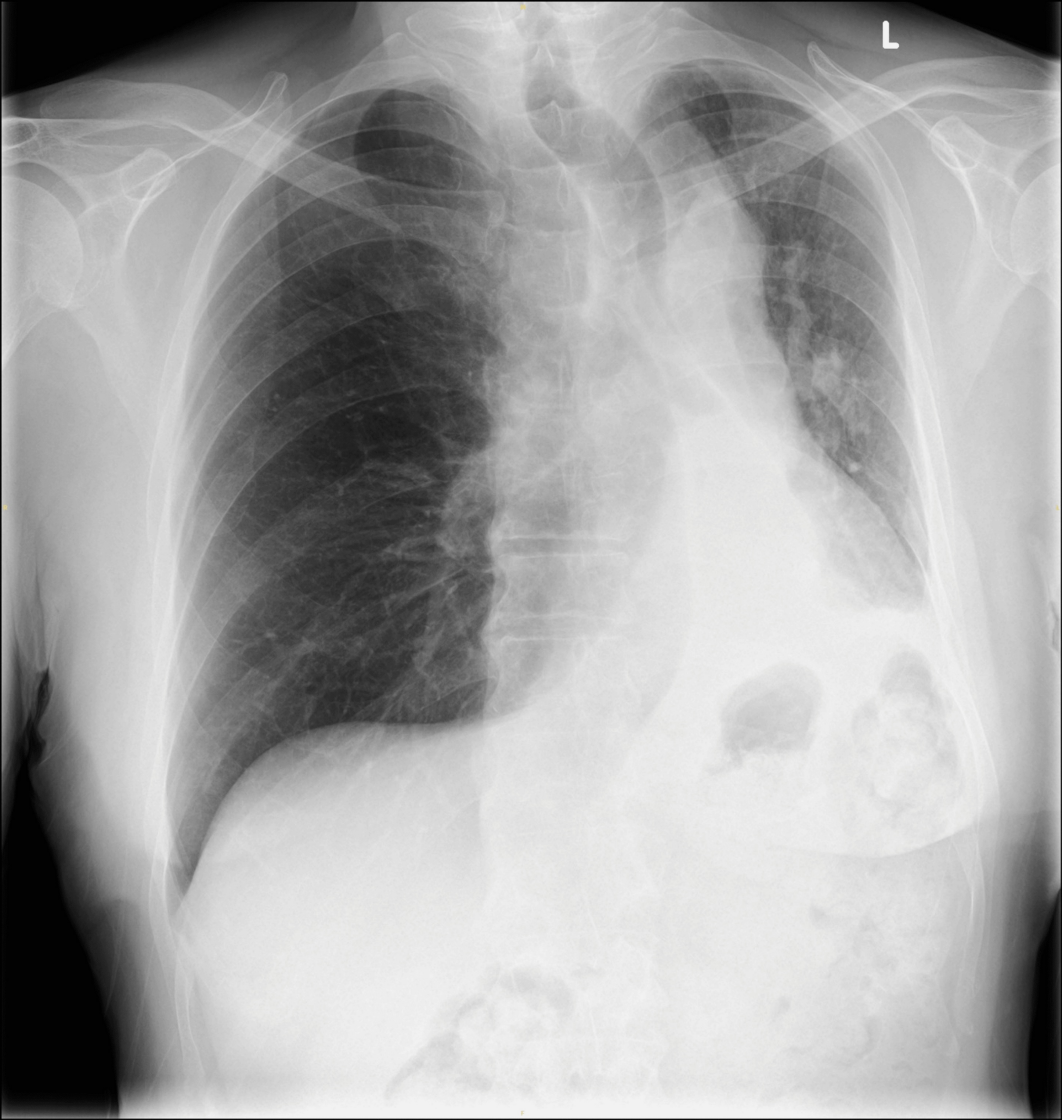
Chest X-ray performed on the day of discharge showing partial left lung re-expansion.
